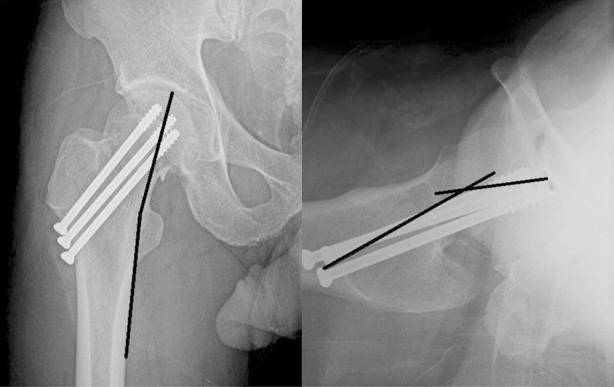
After fixation of femoral neck fracture. We measured trabecular-shaft angle on Anteroposterior radiograph and Anterior angulation on lateral view.
